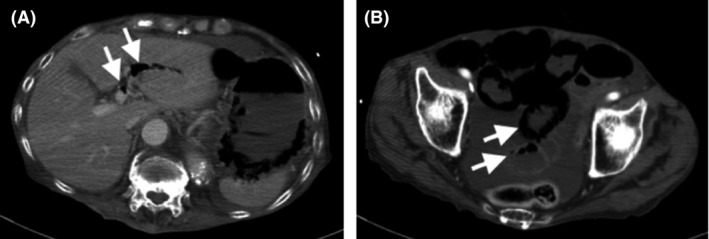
Whole body computed tomography scan of an 84‐year‐old man with self‐inflicted stab wounds to the neck and abdomen, taken when the patient presented with vomiting. A, Image of the upper abdomen. Arrows indicate portal vein gas. B, Image of the lower abdomen. Arrows indicate pneumatosis cystoides intestinalis.
